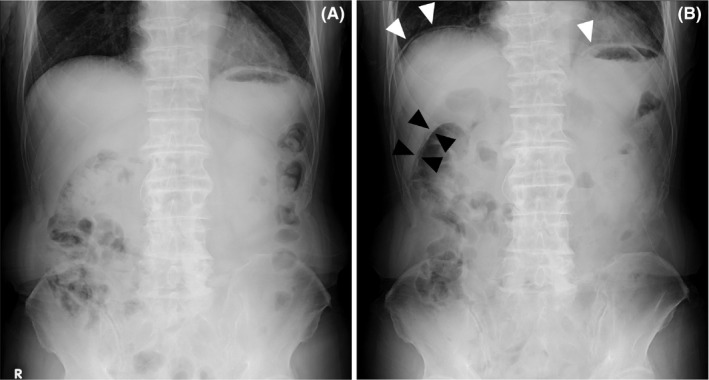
(A) Abdominal radiograph at the first presentation. (B) Abdominal radiograph demonstrating a “double‐wall sign” in the transverse colon in the right subhepatic space (black arrowheads), which could not have been detected in the preceding radiograph (A) during the first presentation, and additional bilateral subdiaphragmatic free air 2 days after the first presentation (white arrowheads)
